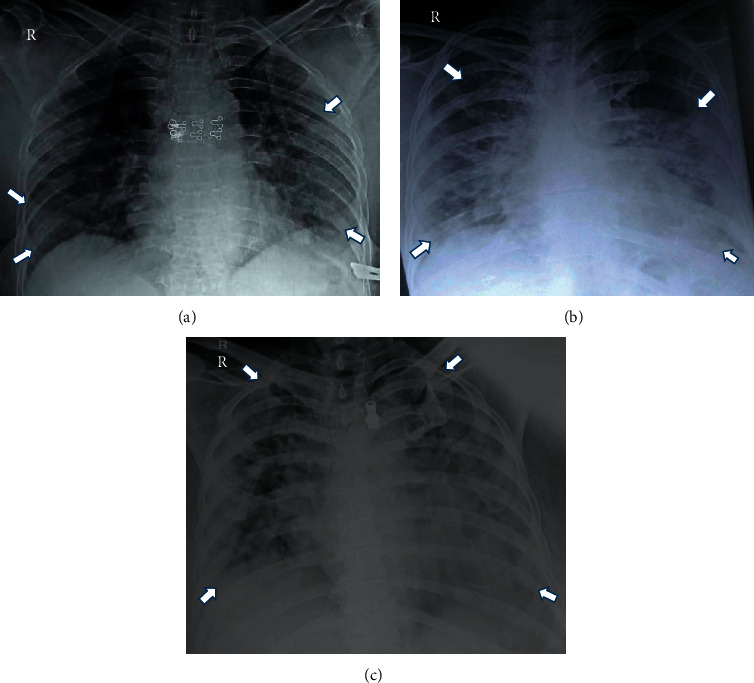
Evolution of chest X-ray findings in a COVID-19 patient with an X-ray scoring system. (a) Day 3: RALE score: 2 + 3 = 5. (b) Day 7: RALE score: 4 + 3 = 7. (c) Day 9: RALE score: 4 + 4 = 8 patient died on day 10.
